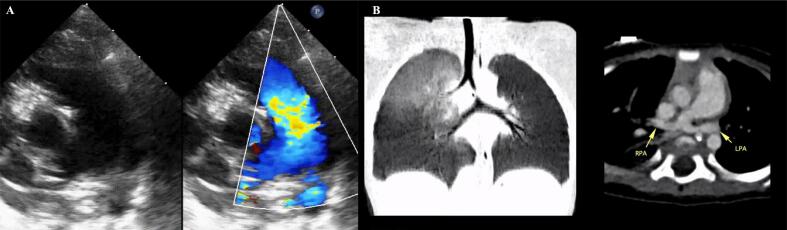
Preoperative assessment. Short axis view echo pre-operative showed LPA originated from RPA as shown in A. Pre-operative CT scan confirmed LPA as shown in B. The LPA can be seen originating from the RPA and wrapping around the distal trachea and right main stem bronchus en route to the left lung.
